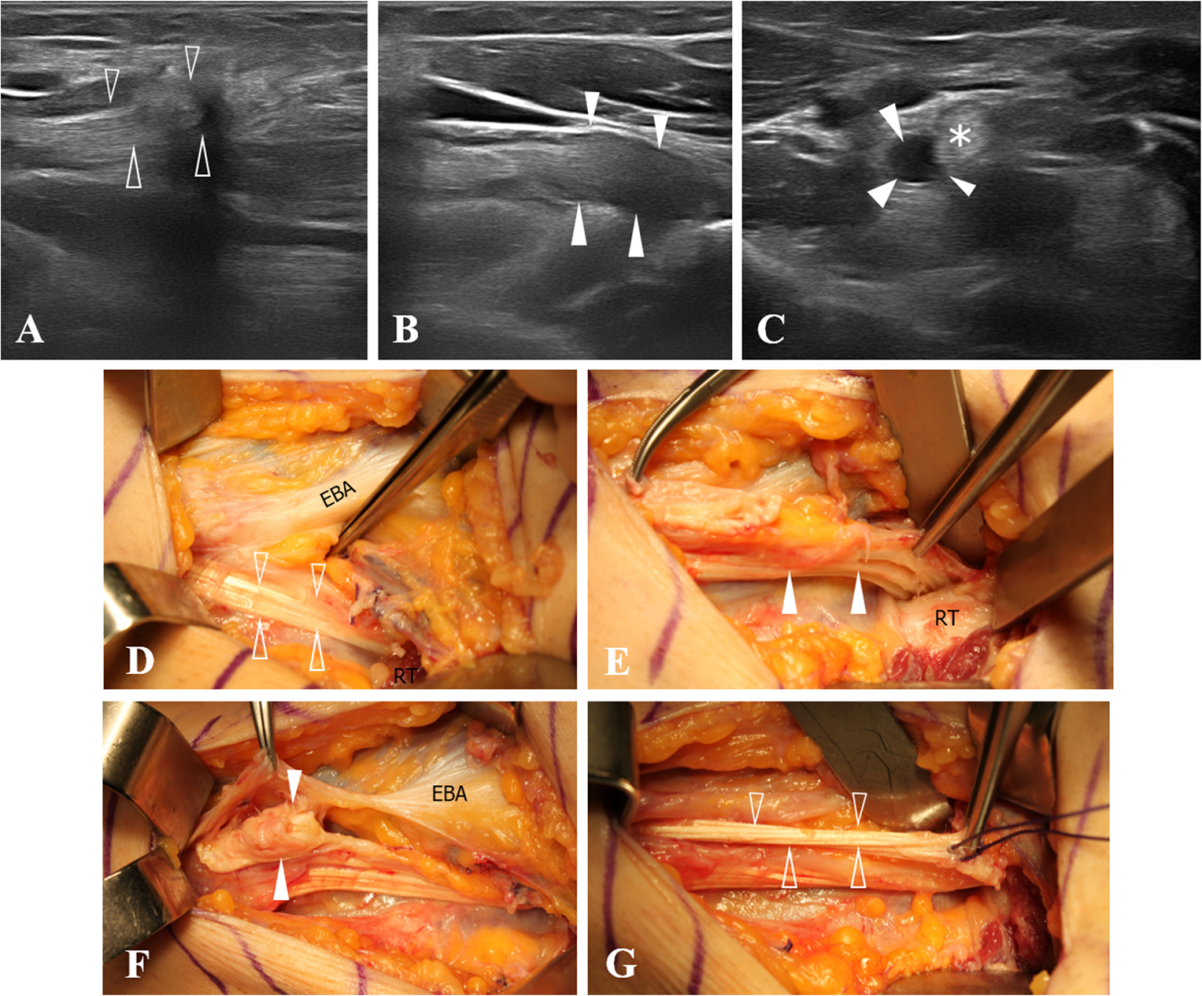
Ultrasound view of a type 2C injury (high-grade partial tear with retraction of one of the DBBT components) and images of surgical confirmation. a In the long-axis view, the DBBT signal disruption and a refraction artefact at the level of the tendon stump indicate a tendon tear (*arrowheads*) affecting the short head component of the DBBT, which has detached and retracted from the radial tuberosity. b However, when slightly shifting the transducer, it is possible to verify that the other DBBT component, corresponding to the long head, remains intact (*arrowheads*). c Short-axis view of a and b. Two oval-shaped elements can clearly be observed. The anechoic image (*arrowheads*) corresponds to the absent, torn and retracted short-head DBBT component (*arrowheads*), whereas the hyperechogenic image corresponds to the preserved long-head DBBT component (*asterisk*). d Some DBBT fibres (*arrowheads*) can be observed, as they are found in their normal path and retain tension. In addition, the external bicipital aponeurosis (*EBA*) can be observed expanding medially to the medial epicondyle muscle mass. e When the DBBT fibres identified in d (*arrowheads*) are pulled with the forceps, their attachment to the radial tuberosity (*RT*) can be verified. f The paratenon has been sectioned at the level of the proximal EBA and the tendon stump corresponding to the torn DBBT short-head component (*arrowheads*) is exposed. g The retracted torn DBBT short-head component can be pulled as far as its footprint on the radial tuberosity (*arrowheads*), thereby verifying the complete rupture of this component
